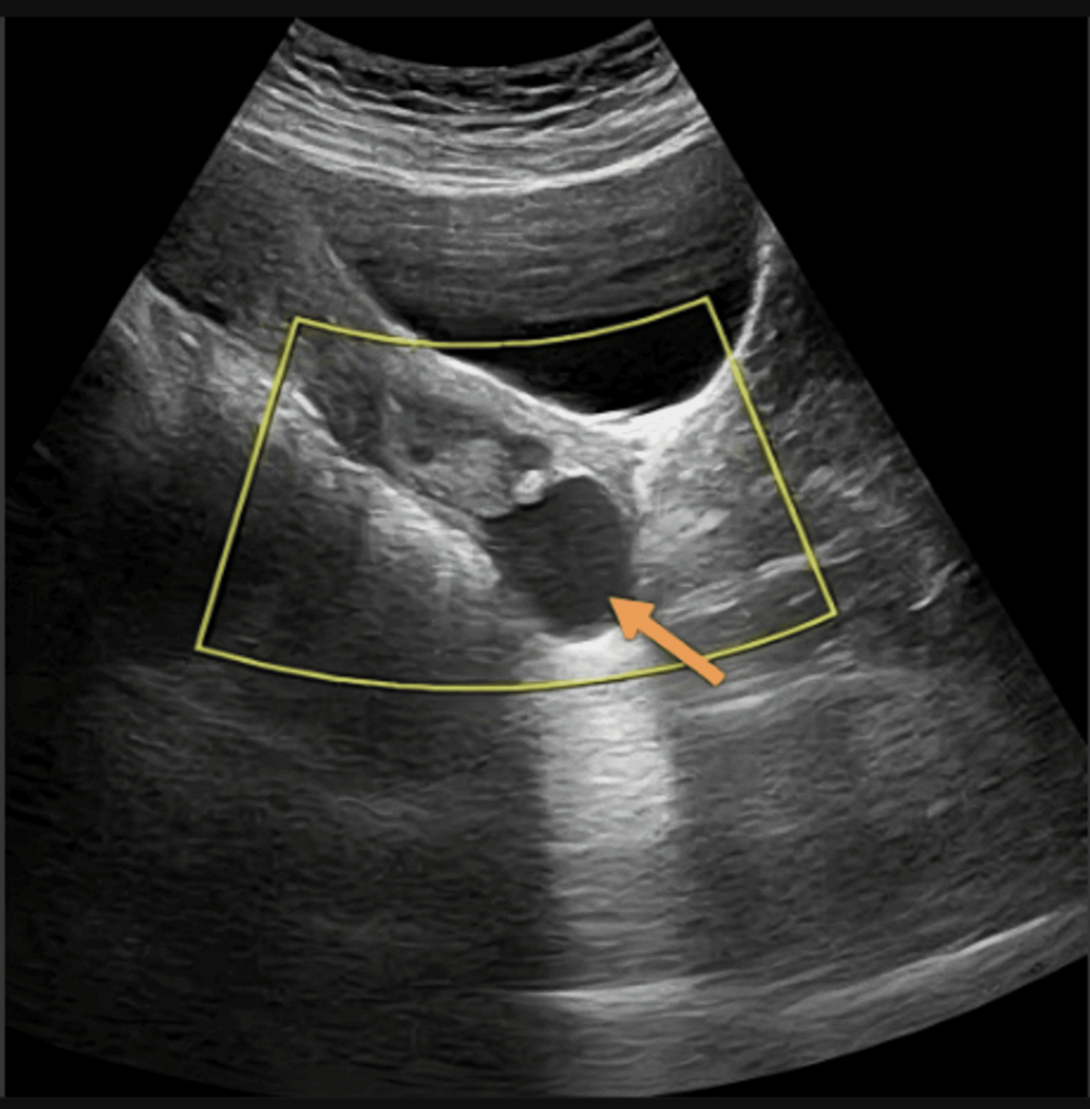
Sagittal color Doppler USG image Color box showing absence of internal vascularity within the lesion (orange arrow).
